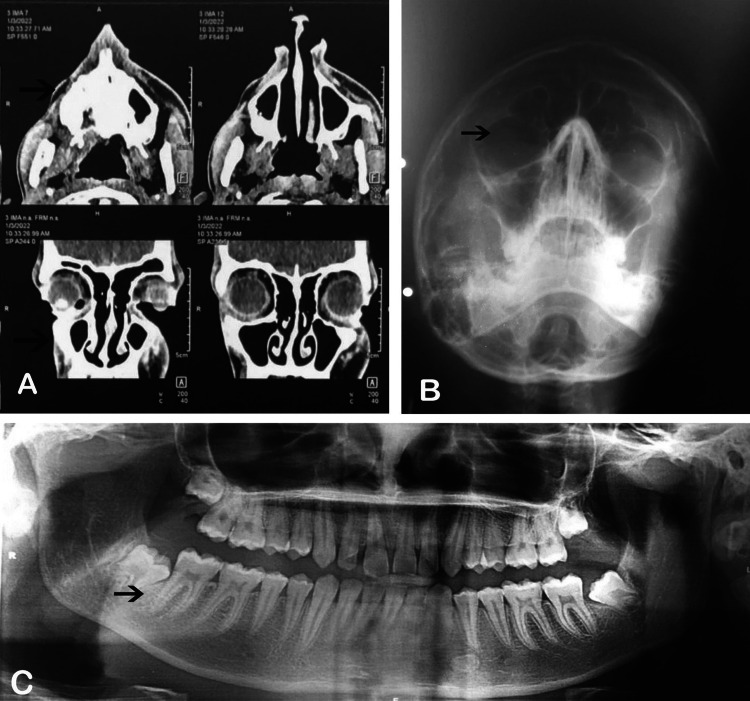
Radiographic features A. CT scan image showing atrophied facial muscles; B. Smaller frontal and maxillary sinus; C. Normal root development in dentition
